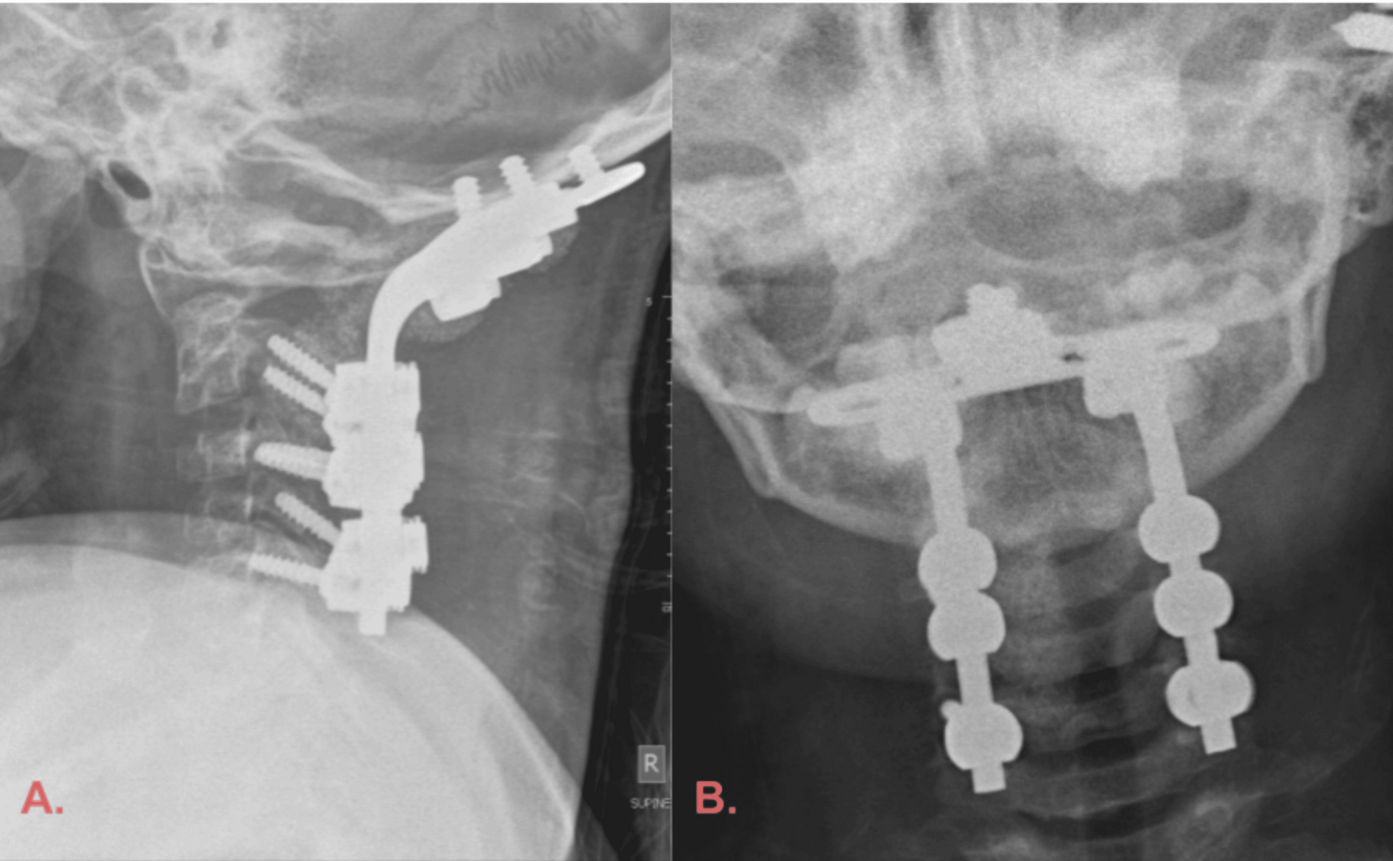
(A) Lateral; (B) Anteroposterior cervical spine X-ray showing the instrumented occipito-cervical fixation.
